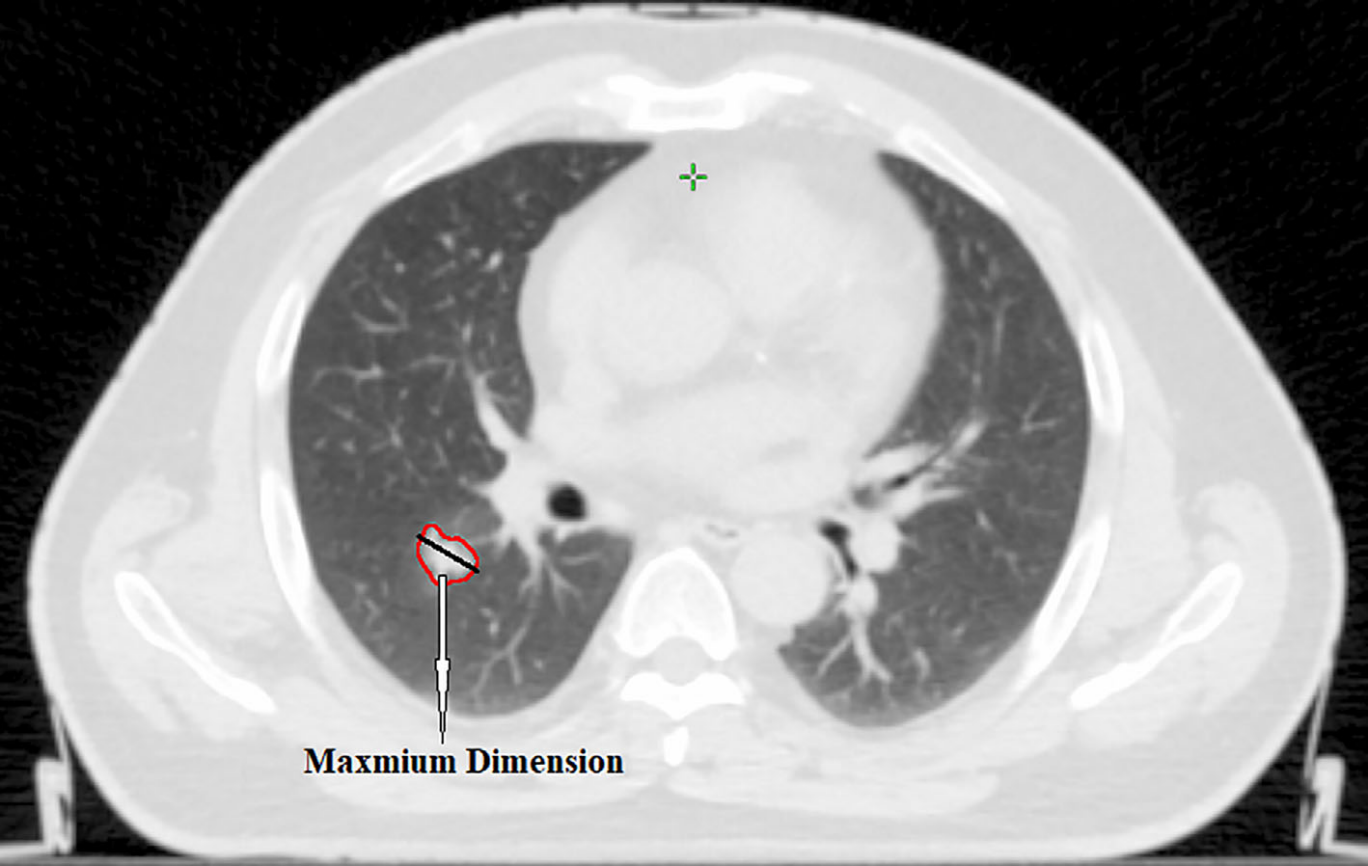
Maximal tumor size as measured on computed tomography (CT) lung windows
